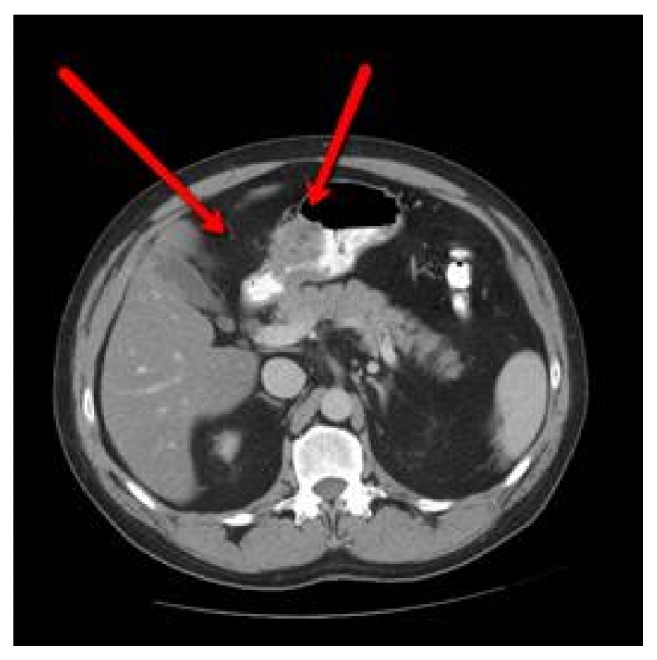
CT scan with IV contrast. Perigastric inflammatory changes are minimal (left arrow). Focal heterogeneous gastric thickening, consistent with residual inflammatory changes (right arrow).
